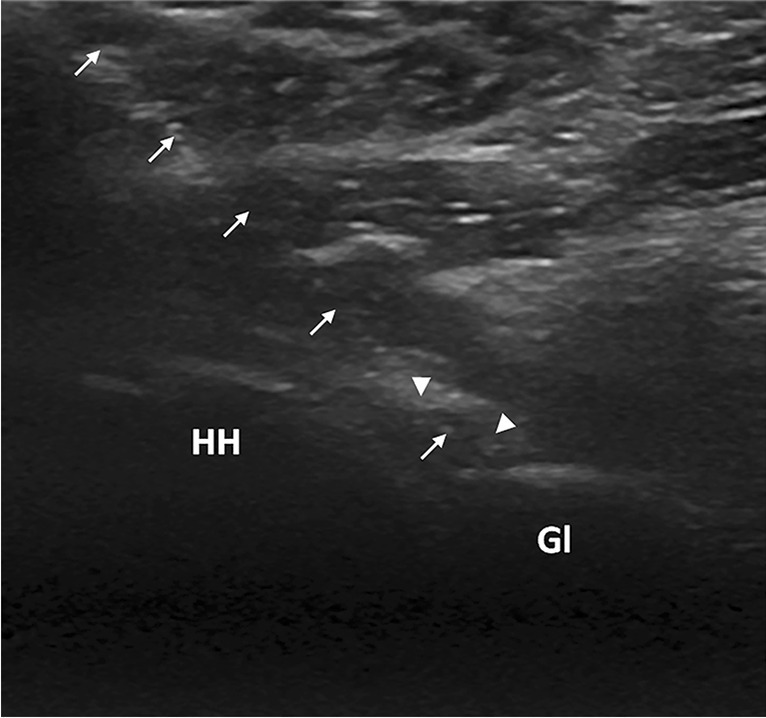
Glenohumeral joint hydrodistension in a 52-year-old female via the posterior approach. Sonographic image showing the needle’s lateral-to-medial course (arrows) ending within a slightly distended posterior joint recess (arrowheads). HH, humeral head; Gl, glenoid
